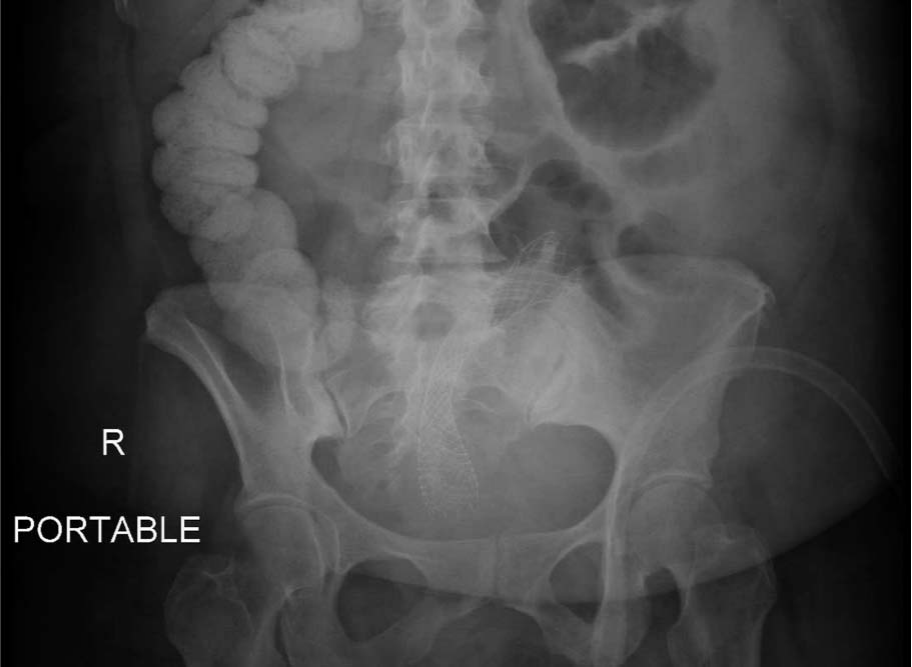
X-ray abdomen showing luminal sigmoid stent.
